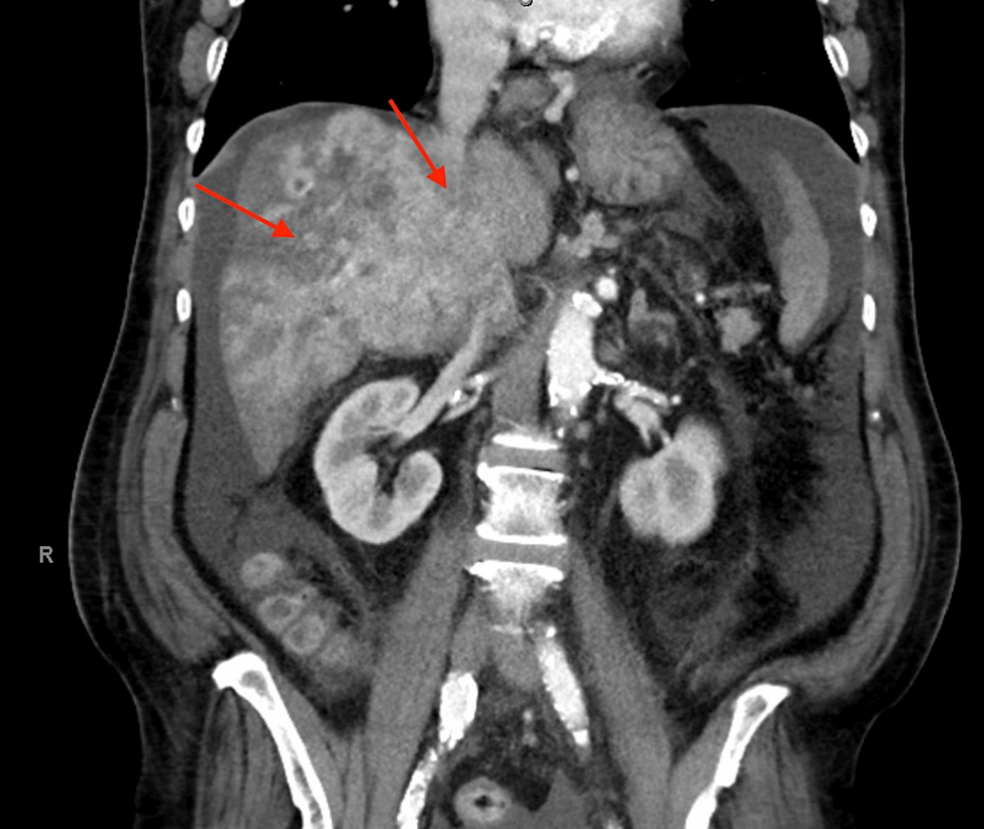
Coronal plane computed tomography (CT) image showing extensive liver metastasis
